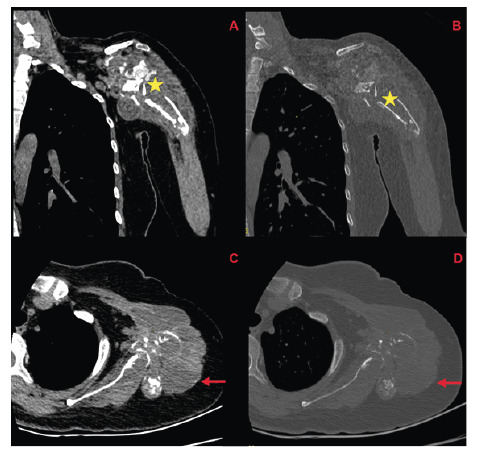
In the patient’s initial shoulder CT scan, bone destruction of the humeral head is observed in both soft tissue and bone windows (yellow asterisks). At this level, the bony cortex is not visualized, and the humeral morphology is severely distorted. In the coronal and axial soft tissue windows, soft tissue masses extending within the muscle planes are identified (red arrows).
